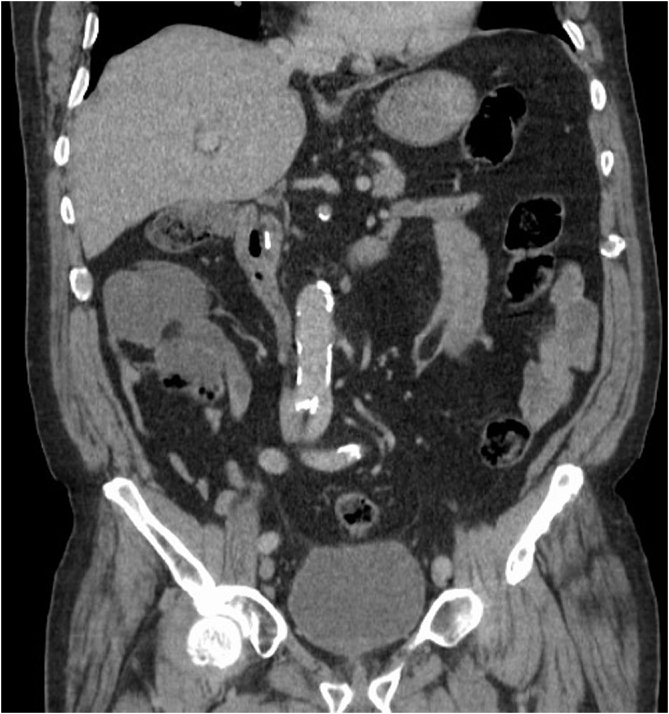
Coronal enhanced CT scan exhibiting a linear metallic density in the CBD consistent with a surgical endoclip.
